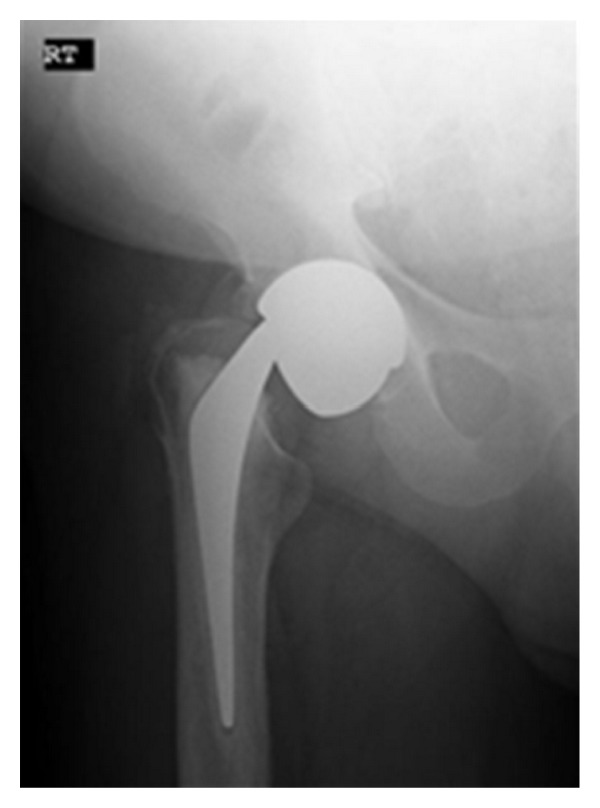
Anteroposterior radiograph demonstrating the fracture of the C-stem at the head-neck junction.
